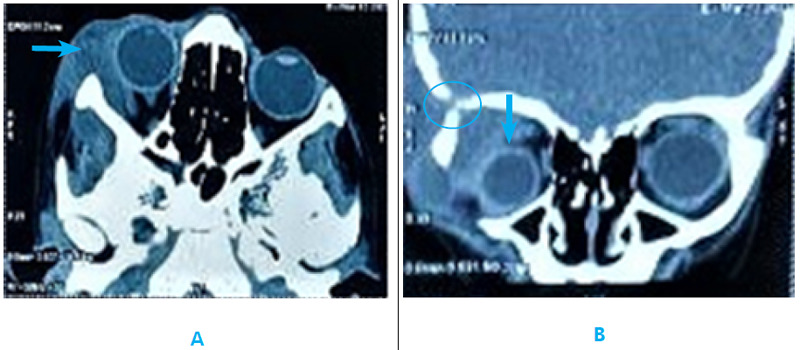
CT orbit (A) axial: right eye medial proptosis with enhancing collection in the right lateral orbital area (arrow) (B) coronal: inferomedial displacement of the right eye (arrow) with superolateral enhancing collection eroding the adjacent orbital bone (circle).
